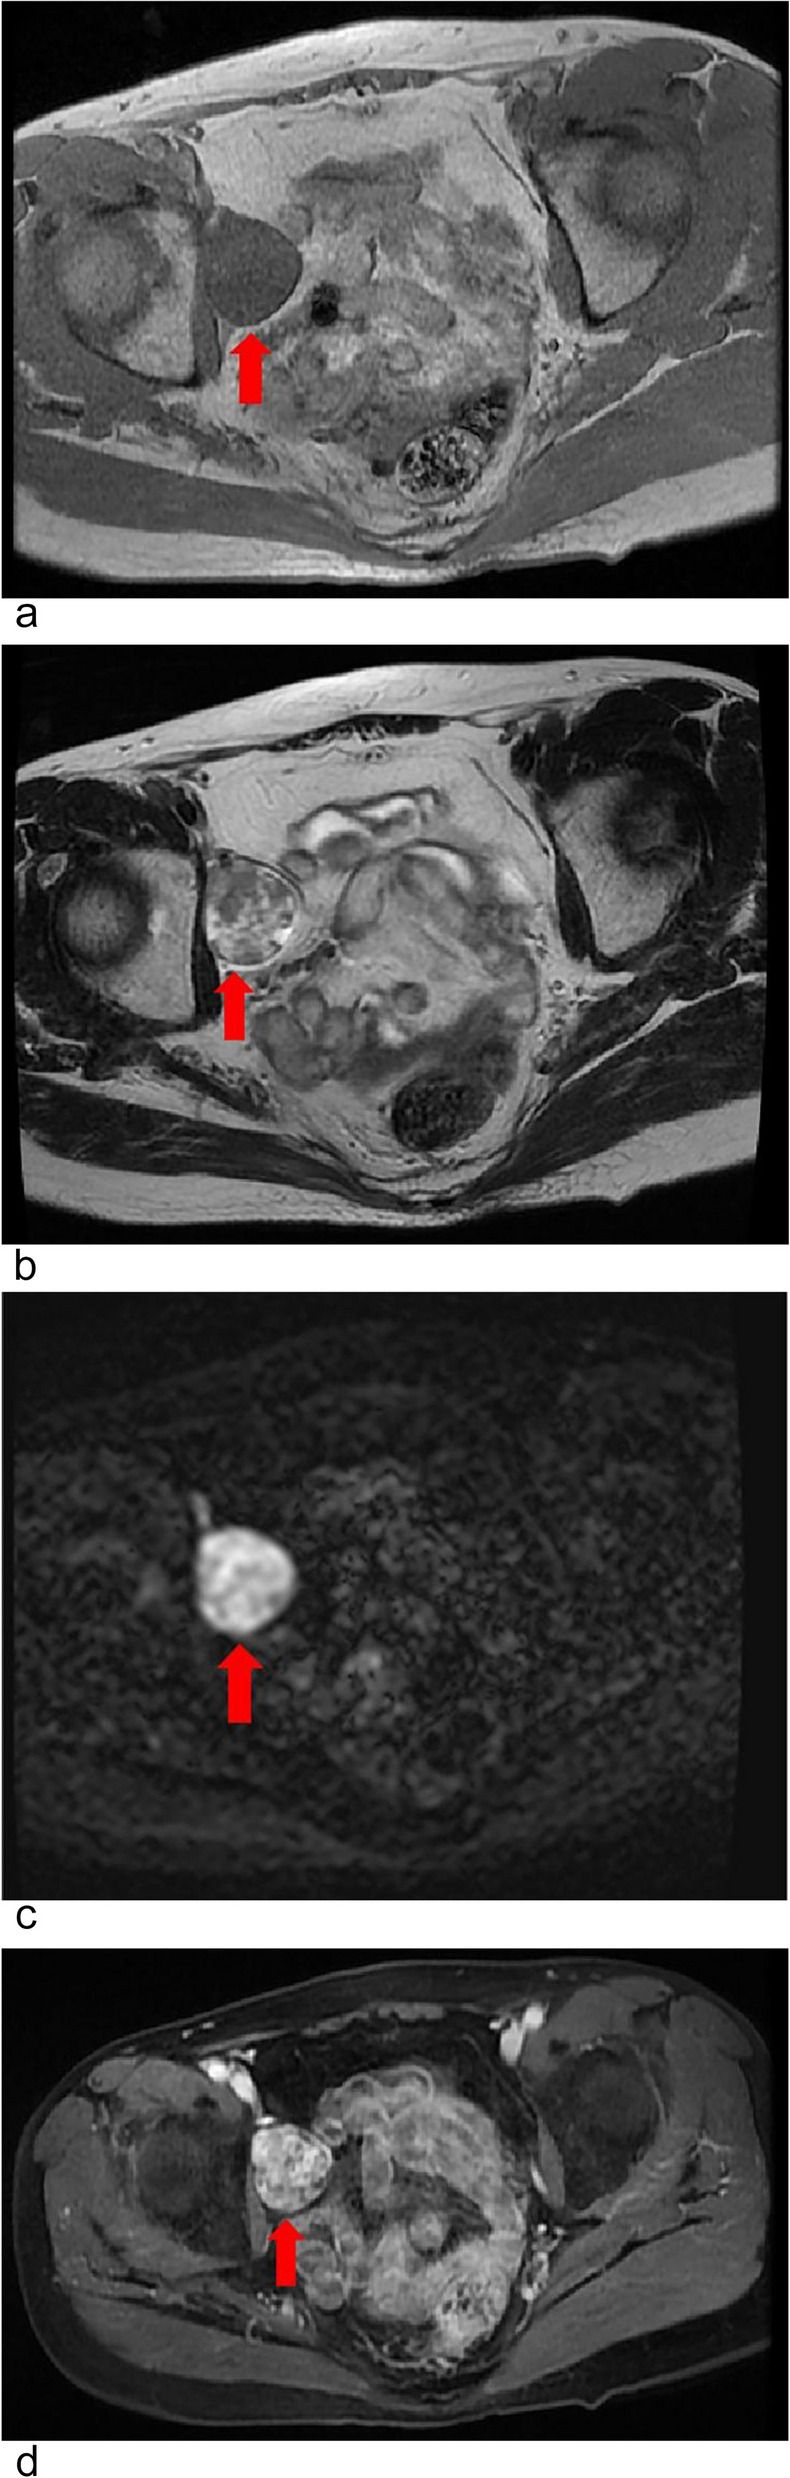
The MRI reveals a round mass in the pelvis (arrow). a Hypointense on T1. b Hyperintense but heterogenous on T2. c Hyperintense on diffusion-weighted imaging. d The solid part of the mass with obvious contrast-enhancement. *MRI *magnetic resonance imaging
